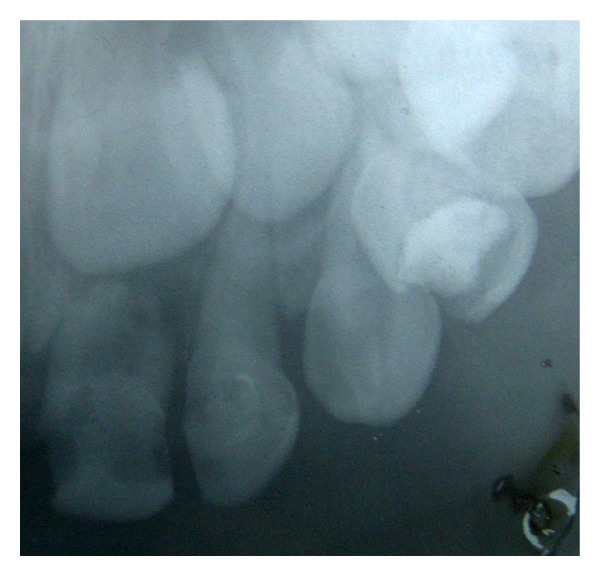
Final radiographic image.
